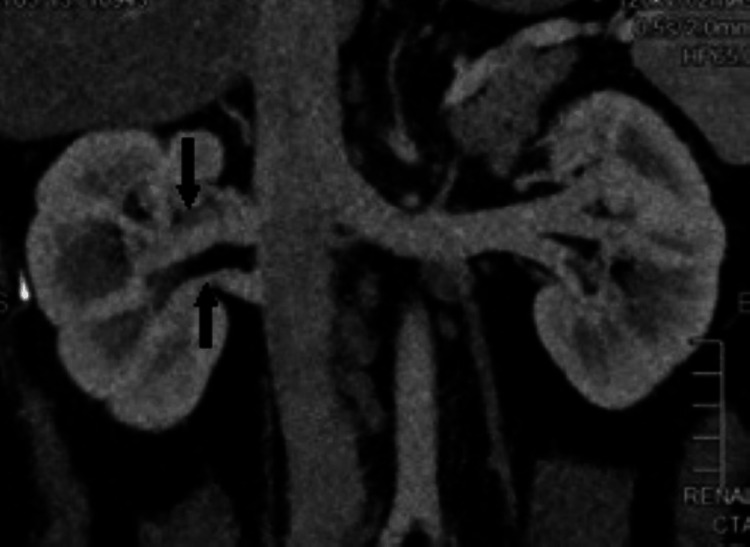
CT angiography image in nephrographic phase shows two right renal veins separately draining to the inferior vena cava.
